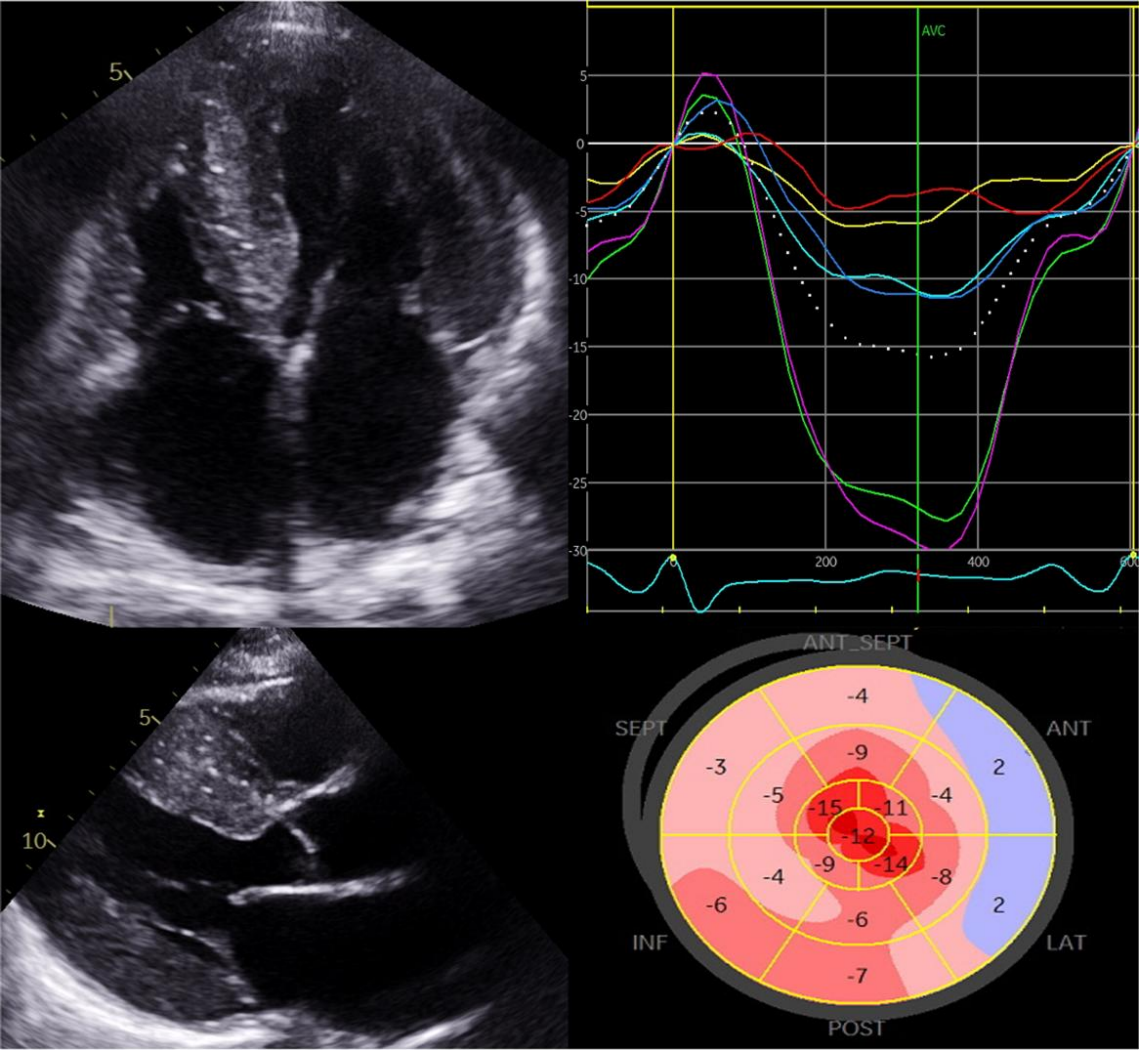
Echocardiographic findings in a patient with advanced transthyretin cardiomyopathy. Parasternal long axis and four-chamber view demonstrating increased biventricular thickening with a ‘speckled’ myocardium (top left panels) with 2D-strain using speckle tracking echocardiography in the same patient (top right panel). Peak systolic strain for individual myocardial segments in the four-chamber view panel and the strain curve samples in each of the corresponding coloured myocardial segments panel can then generate a longitudinal strain map (bottom). The characteristic basal to apical gradient of impaired longitudinal function is observed here.
